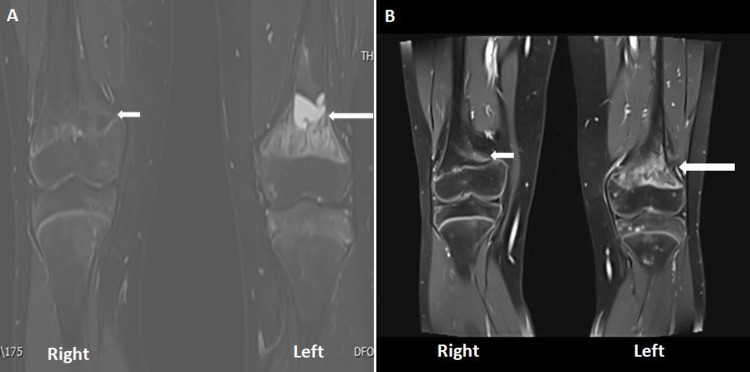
Gadolinium-enhanced MRI of the knee. A: Selected STIR sequence: it shows high signal intensity lesions in the distal metaphysics of both femurs, more severely involving the left side.  B: Gadolinium-enhanced sequence demonstrating heterogeneous intense enhancement on the left side in the affected metaphyseal region of the left knee (long white arrow) and mild enhancement on the right side (short white arrow). STIR, short tau inversion recovery
